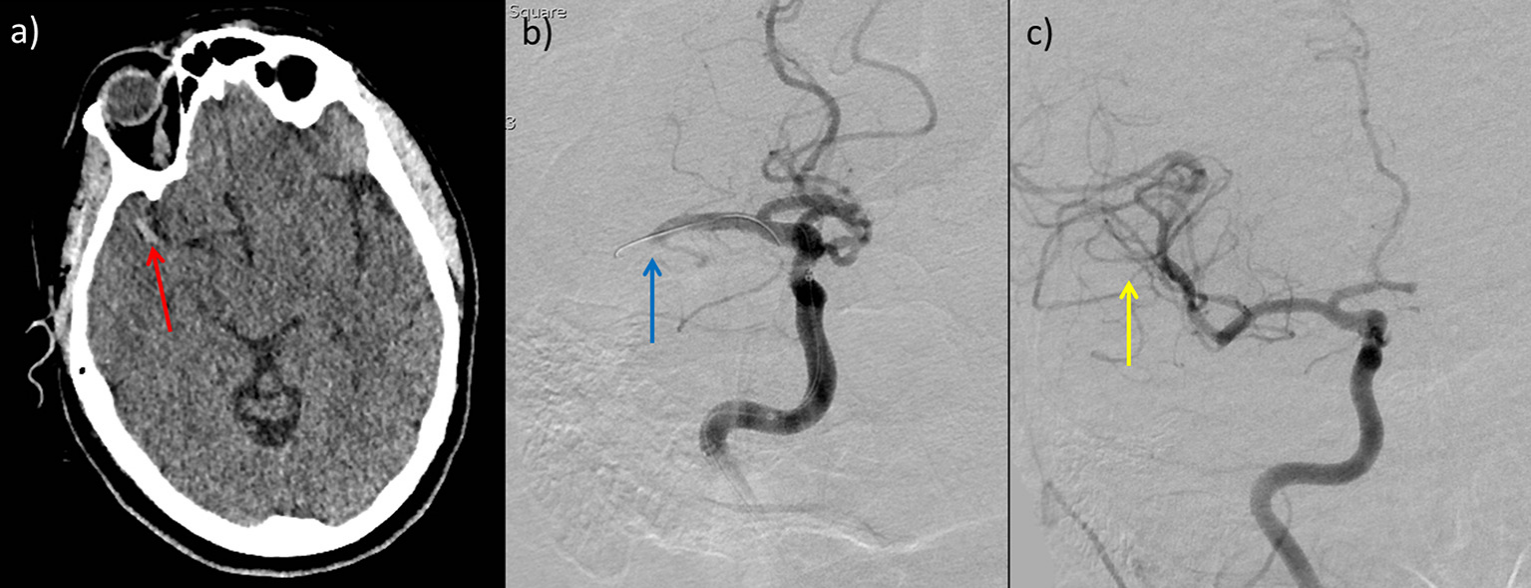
Plain CT scan of head (a) and prethrombectomy (b) and post-thrombectomy (c) digital subtraction angiograms in a 49-year-old woman with sudden onset left hemiparesis and confusion. Plain CT scan of head shows hyperdense clot in the right middle cerebral artery (red arrow) and early perisylvian loss of grey–white matter differentiation. Prethrombectomy digital subtraction angiogram shows occluded right proximal middle cerebral artery (blue arrow). The catheter is visible passing through the occlusion. Post-procedure imaging shows good filling of all middle cerebral artery branches (yellow arrow). There was complete resolution of neurological signs and symptoms following aspiration thrombectomy.
